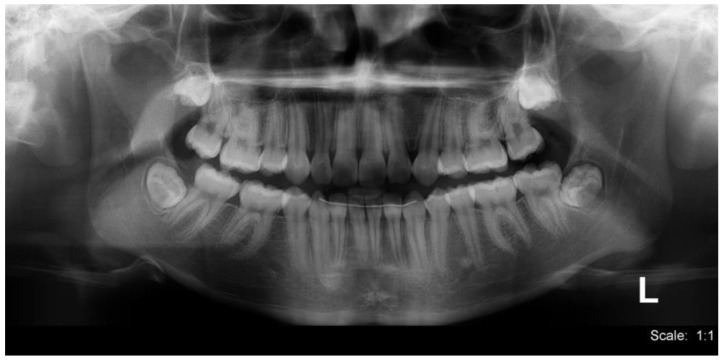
Panoramic X-ray. 2023, 24 months after the second surgical removal. Permanent dentition. Third molars in intraosseous evolution. Anterior mandibular splinting.
